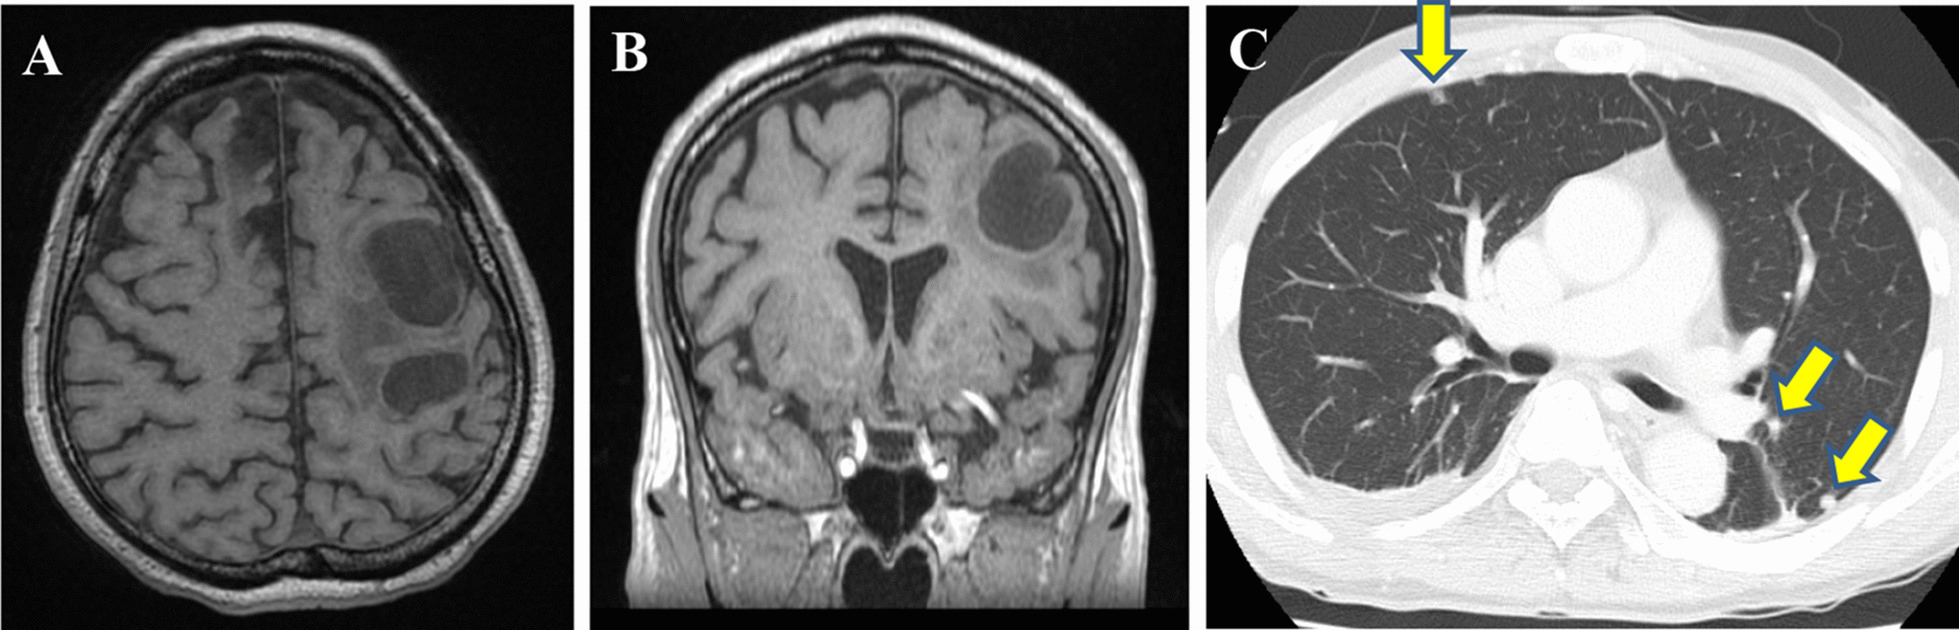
Radiological images of brain and lung metastases at 8 months after operation (a, b) Magnetic resonance imaging revealed brain metastasis at the left parietal lobe with two cystic lesions (a: axial view and b: coronal view). c Computed tomography revealed multiple metastases in both sides of the lungs (yellow arrows)
